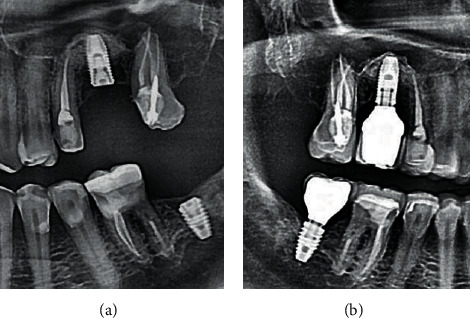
X-ray control: (a) implant integration after 16 weeks; (b) bone remodeling around the implant neck 4 weeks after placement of the platform switching prosthesis.
